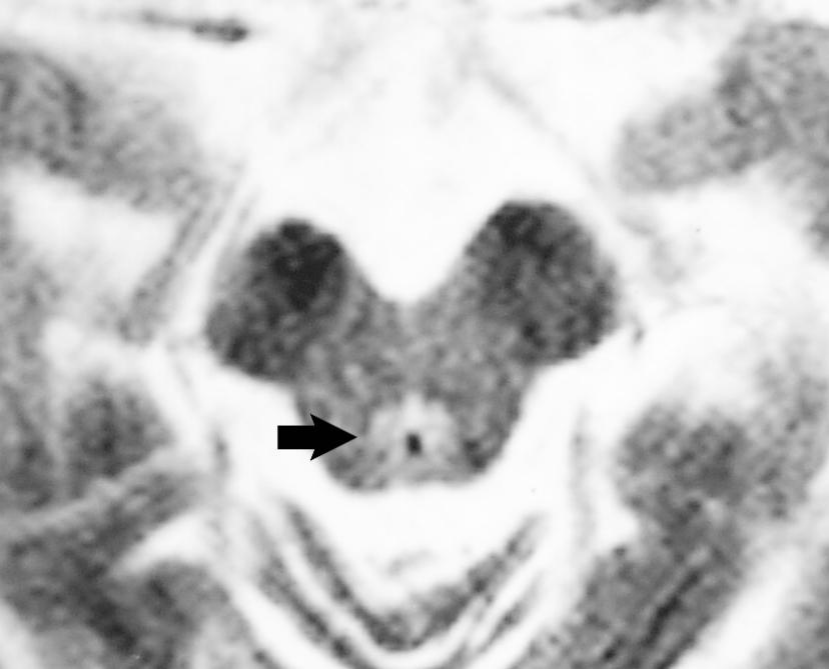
Brain axial T2W magnetic resonance imaging at the level of the midbrain. High signal around the Aqueduct of Sylvius (arrow).
